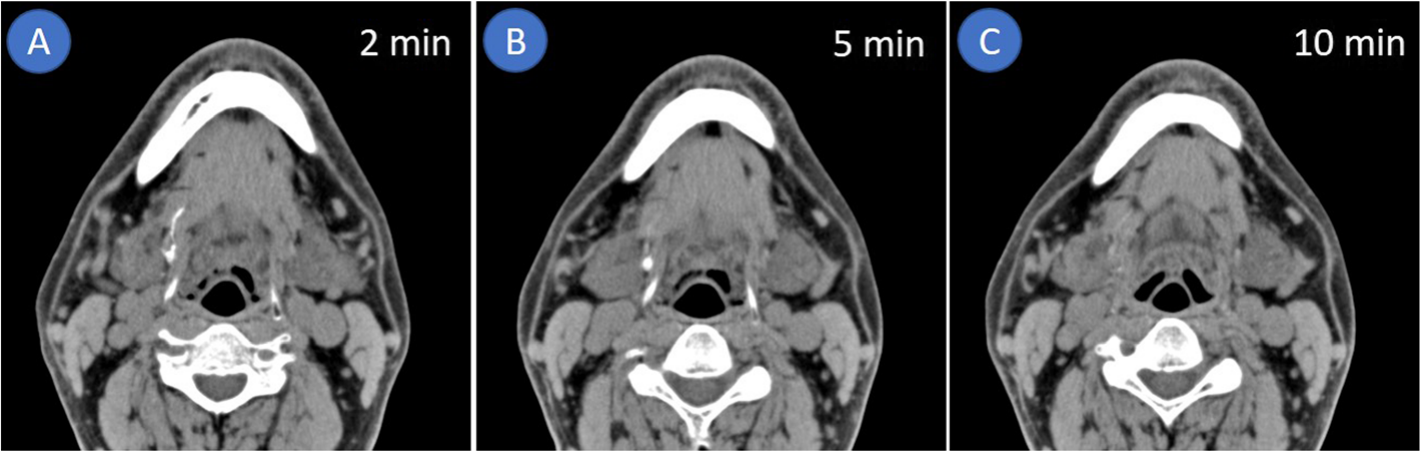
CT lymphography (axial image). a: Sentinel lymph node and lymphatic visualized 2 min after local injection of iopamidol. b: Sentinel lymph node clearly enhanced 5 min after contrast injection, with disappearance of the lymphatic vessel. c: Disappearance of both the sentinel lymph node and lymphatic vessel 10 min after contrast injection
